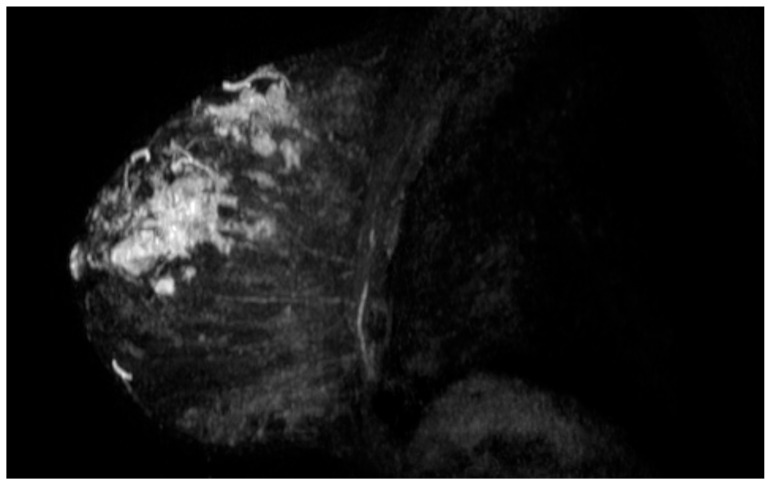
Magnetic resonance imaging revealing the 10 cm ductal carcinoma in the right breast.
